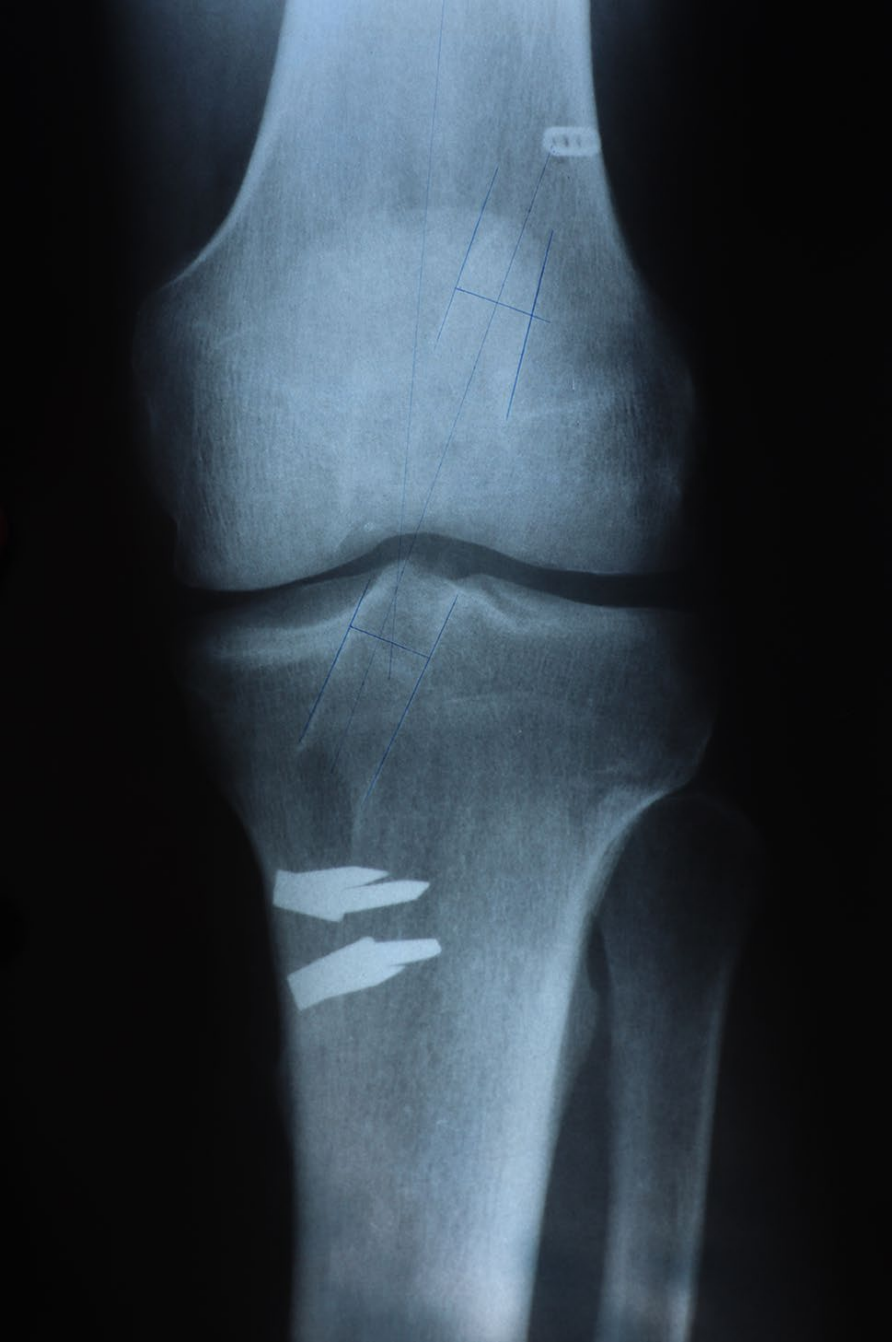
Tunnel widening on the AP X-ray, using HT graft
